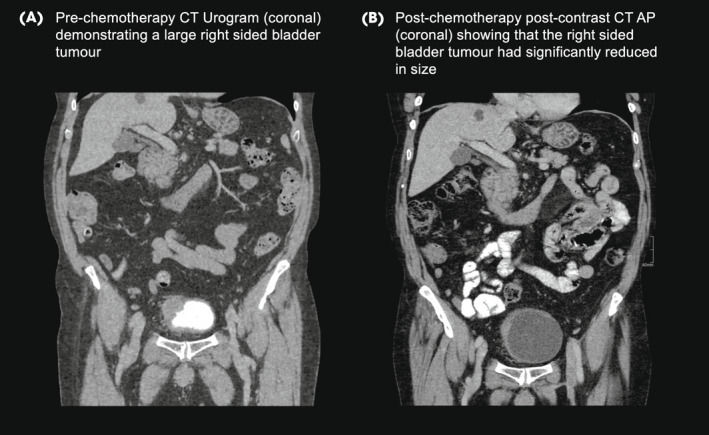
Pre and post‐neoadjuvant chemotherapy computed tomography (CT) appearances of the right sided muscle invasive bladder tumour.
